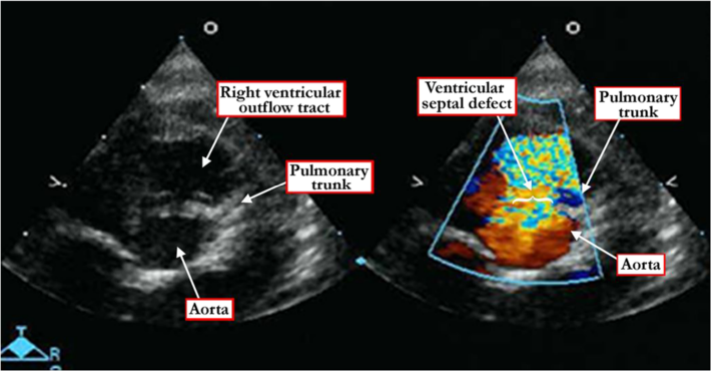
The short axis echocardiographic cut (left panel) demonstrates the fibrous continuity between the leaflets of the aortic and pulmonary valves that identifies the defect as being doubly committed and juxta-arterial. The right panel shows the Doppler color flow map of the same defect.
